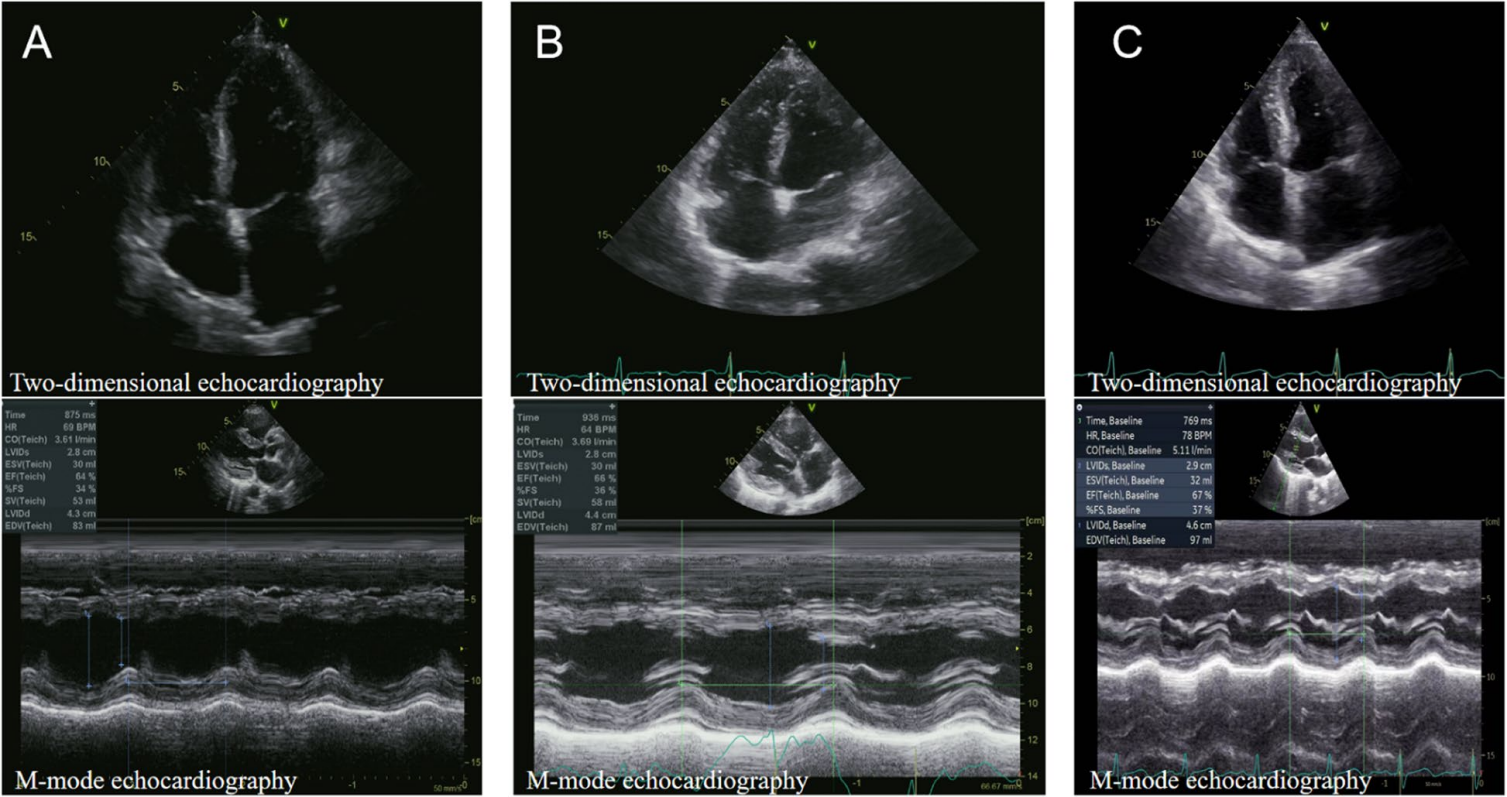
Two-dimensional and M-mode echocardiography at 2-month follow-up (A); 4-month follow-up and 6-month follow-up (C)
